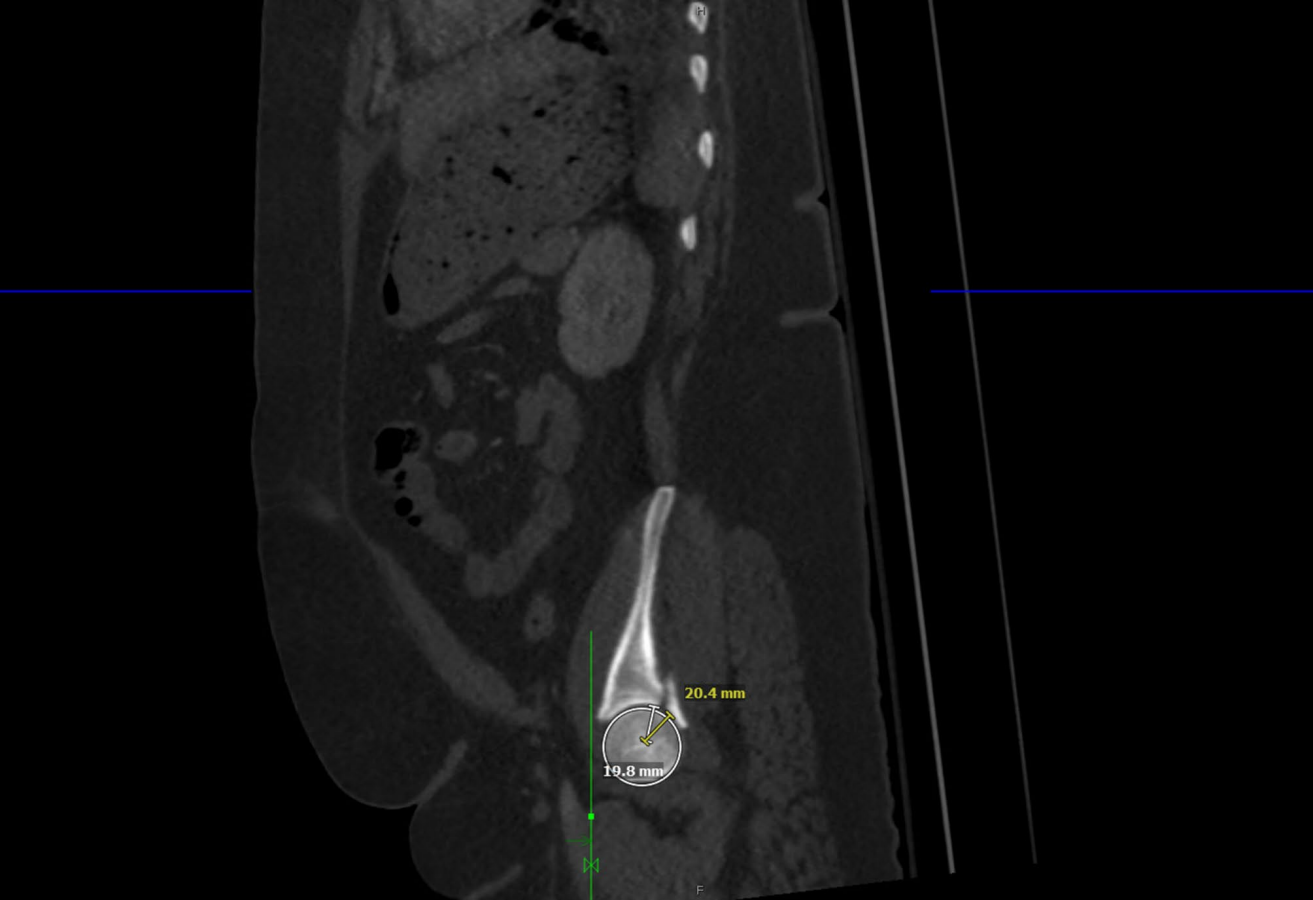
Step-off measurement in sagittal computed tomography axis
